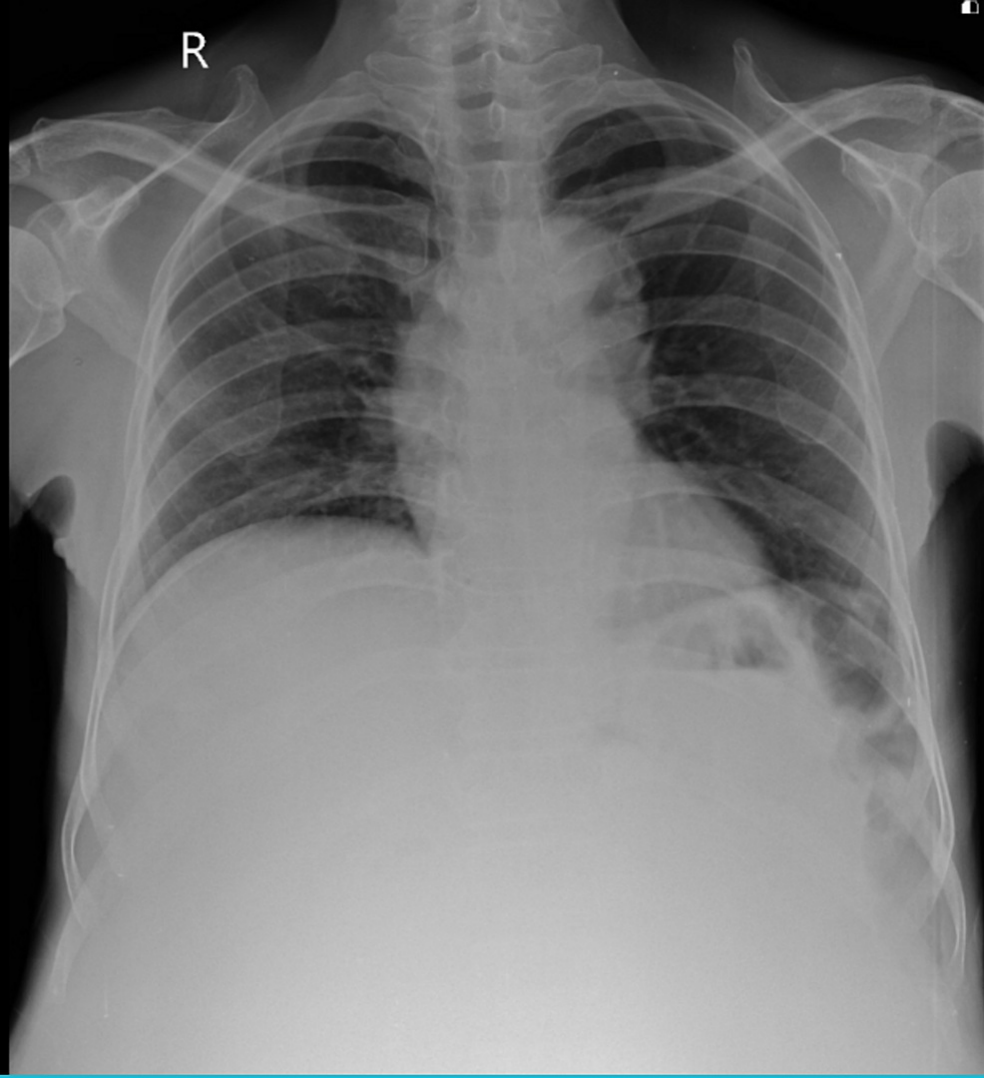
X-ray showing pushed-up diaphragm and obliterated costophrenic angle
